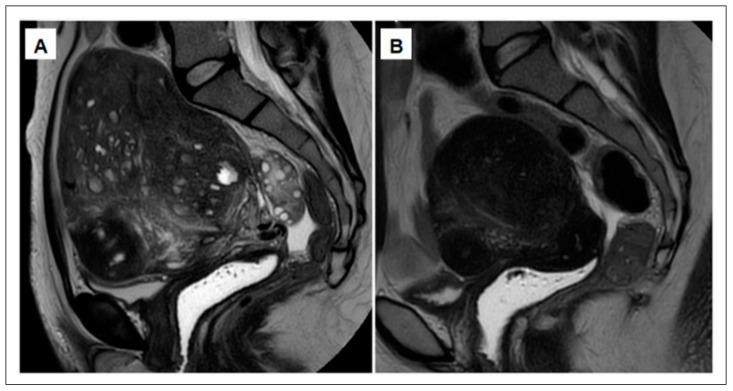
(A) MRI showing a very large uterus, consistent with severe full-thickness adenomyosis. (B) After a 12-week course of GnRH antagonist (daily dose of 200 mg linzagolix), a significant reduction is observed in both uterine size and adenomyotic foci (adapted from [73]).
